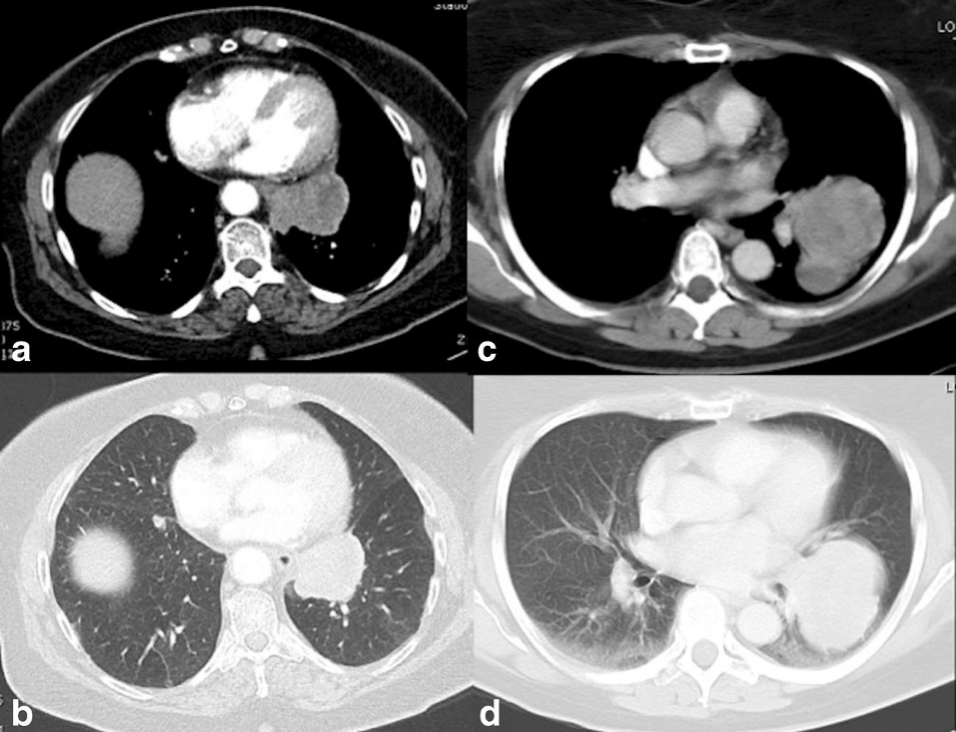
CT images of primary pulmonary sarcomas. A 67-year-old woman (a and b) with synovial sarcoma in the left lower lobe, presenting irregular shape, smooth margins and heterogeneous enhancement. A 61-year-old woman (c and d) with leiomyosarcoma, showing irregular shape, lobulated margins and heterogeneous enhancement
